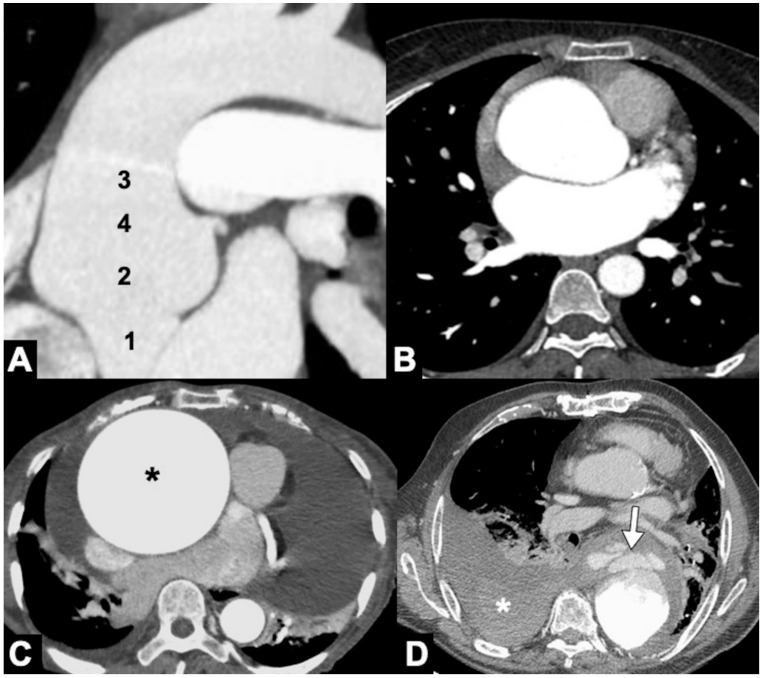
CTA of thoracic aorta aneurysms. (A) Marfan syndrome, a multisystem connective tissue disease caused by a defect in the protein fibrillin 1, and annuloaortic ectasia in a 42-year-old man. Sagittal MIP reconstruction image shows a proximal dilatation of the aortic anulus (1), sinuses of Valsalva (2) along with effacement of the STJ (4) producing a pear-shaped ascending aorta (3). (B) Axial CTA image shows sinuses ectasia in the same patient. In Marfan syndrome, a cut-off value of 5 cm of the ascending aorta diameter is recommended for surgical repair. (C) Axial CTA image shows a 12 cm ascending aorta aneurysm (asterisk) and a large mediastinal effusion. The risk of rupture of TAAs increases with size of the aneurysm according to Laplace’s law. (D) Axial CTA image shows a ruptured atherosclerotic aneurysm of the descending thoracic aorta. Note the high-attenuation fluid in the right pleural space, representing acute hemothorax (asterisk), and contrast medium extravasation from the aortic lumen (arrow).
